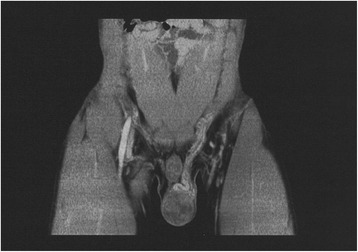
Computed tomography findings in the patient. A partially enhanced localized tumor was detected in his left testis, with no distant metastasis or lymph node swelling
